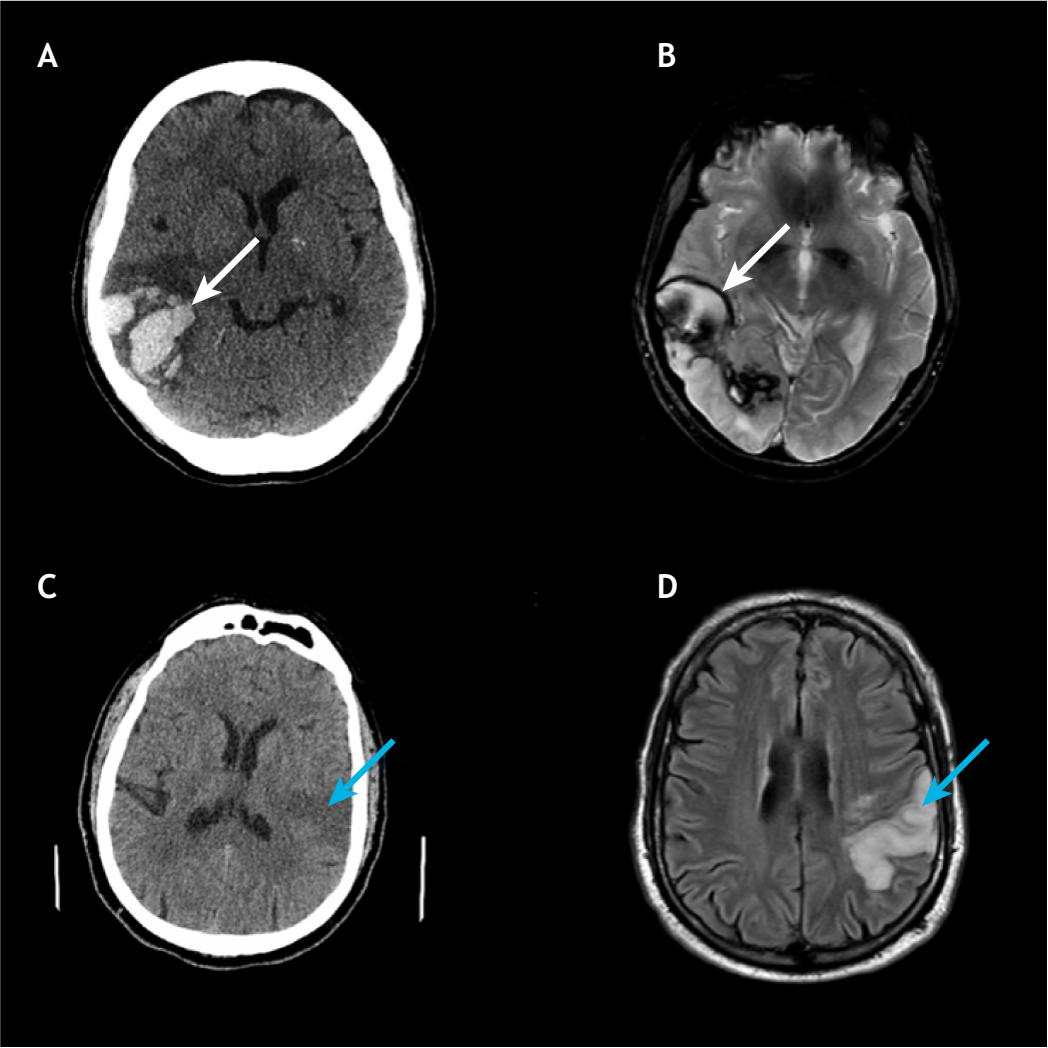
CT versus MRI scans for detecting stroke. (A,B) A haemorrhage can be seen clearly in the CT scan of a patient (A; white arrow), whereas it is less evident in an MRI scan (B; white arrow). (C,D) Whereas an ischaemic infarct is only faintly visible in the CT scan of a patient (C; blue arrow), it is clearly visible in an MRI scan (D; blue arrow). Images reproduced from Heit et al. (2017) and Rajdev et al. (2020) under the terms of the CC-BY 4.0 license. CT, computed tomography; MRI, magnetic resonance imaging.
